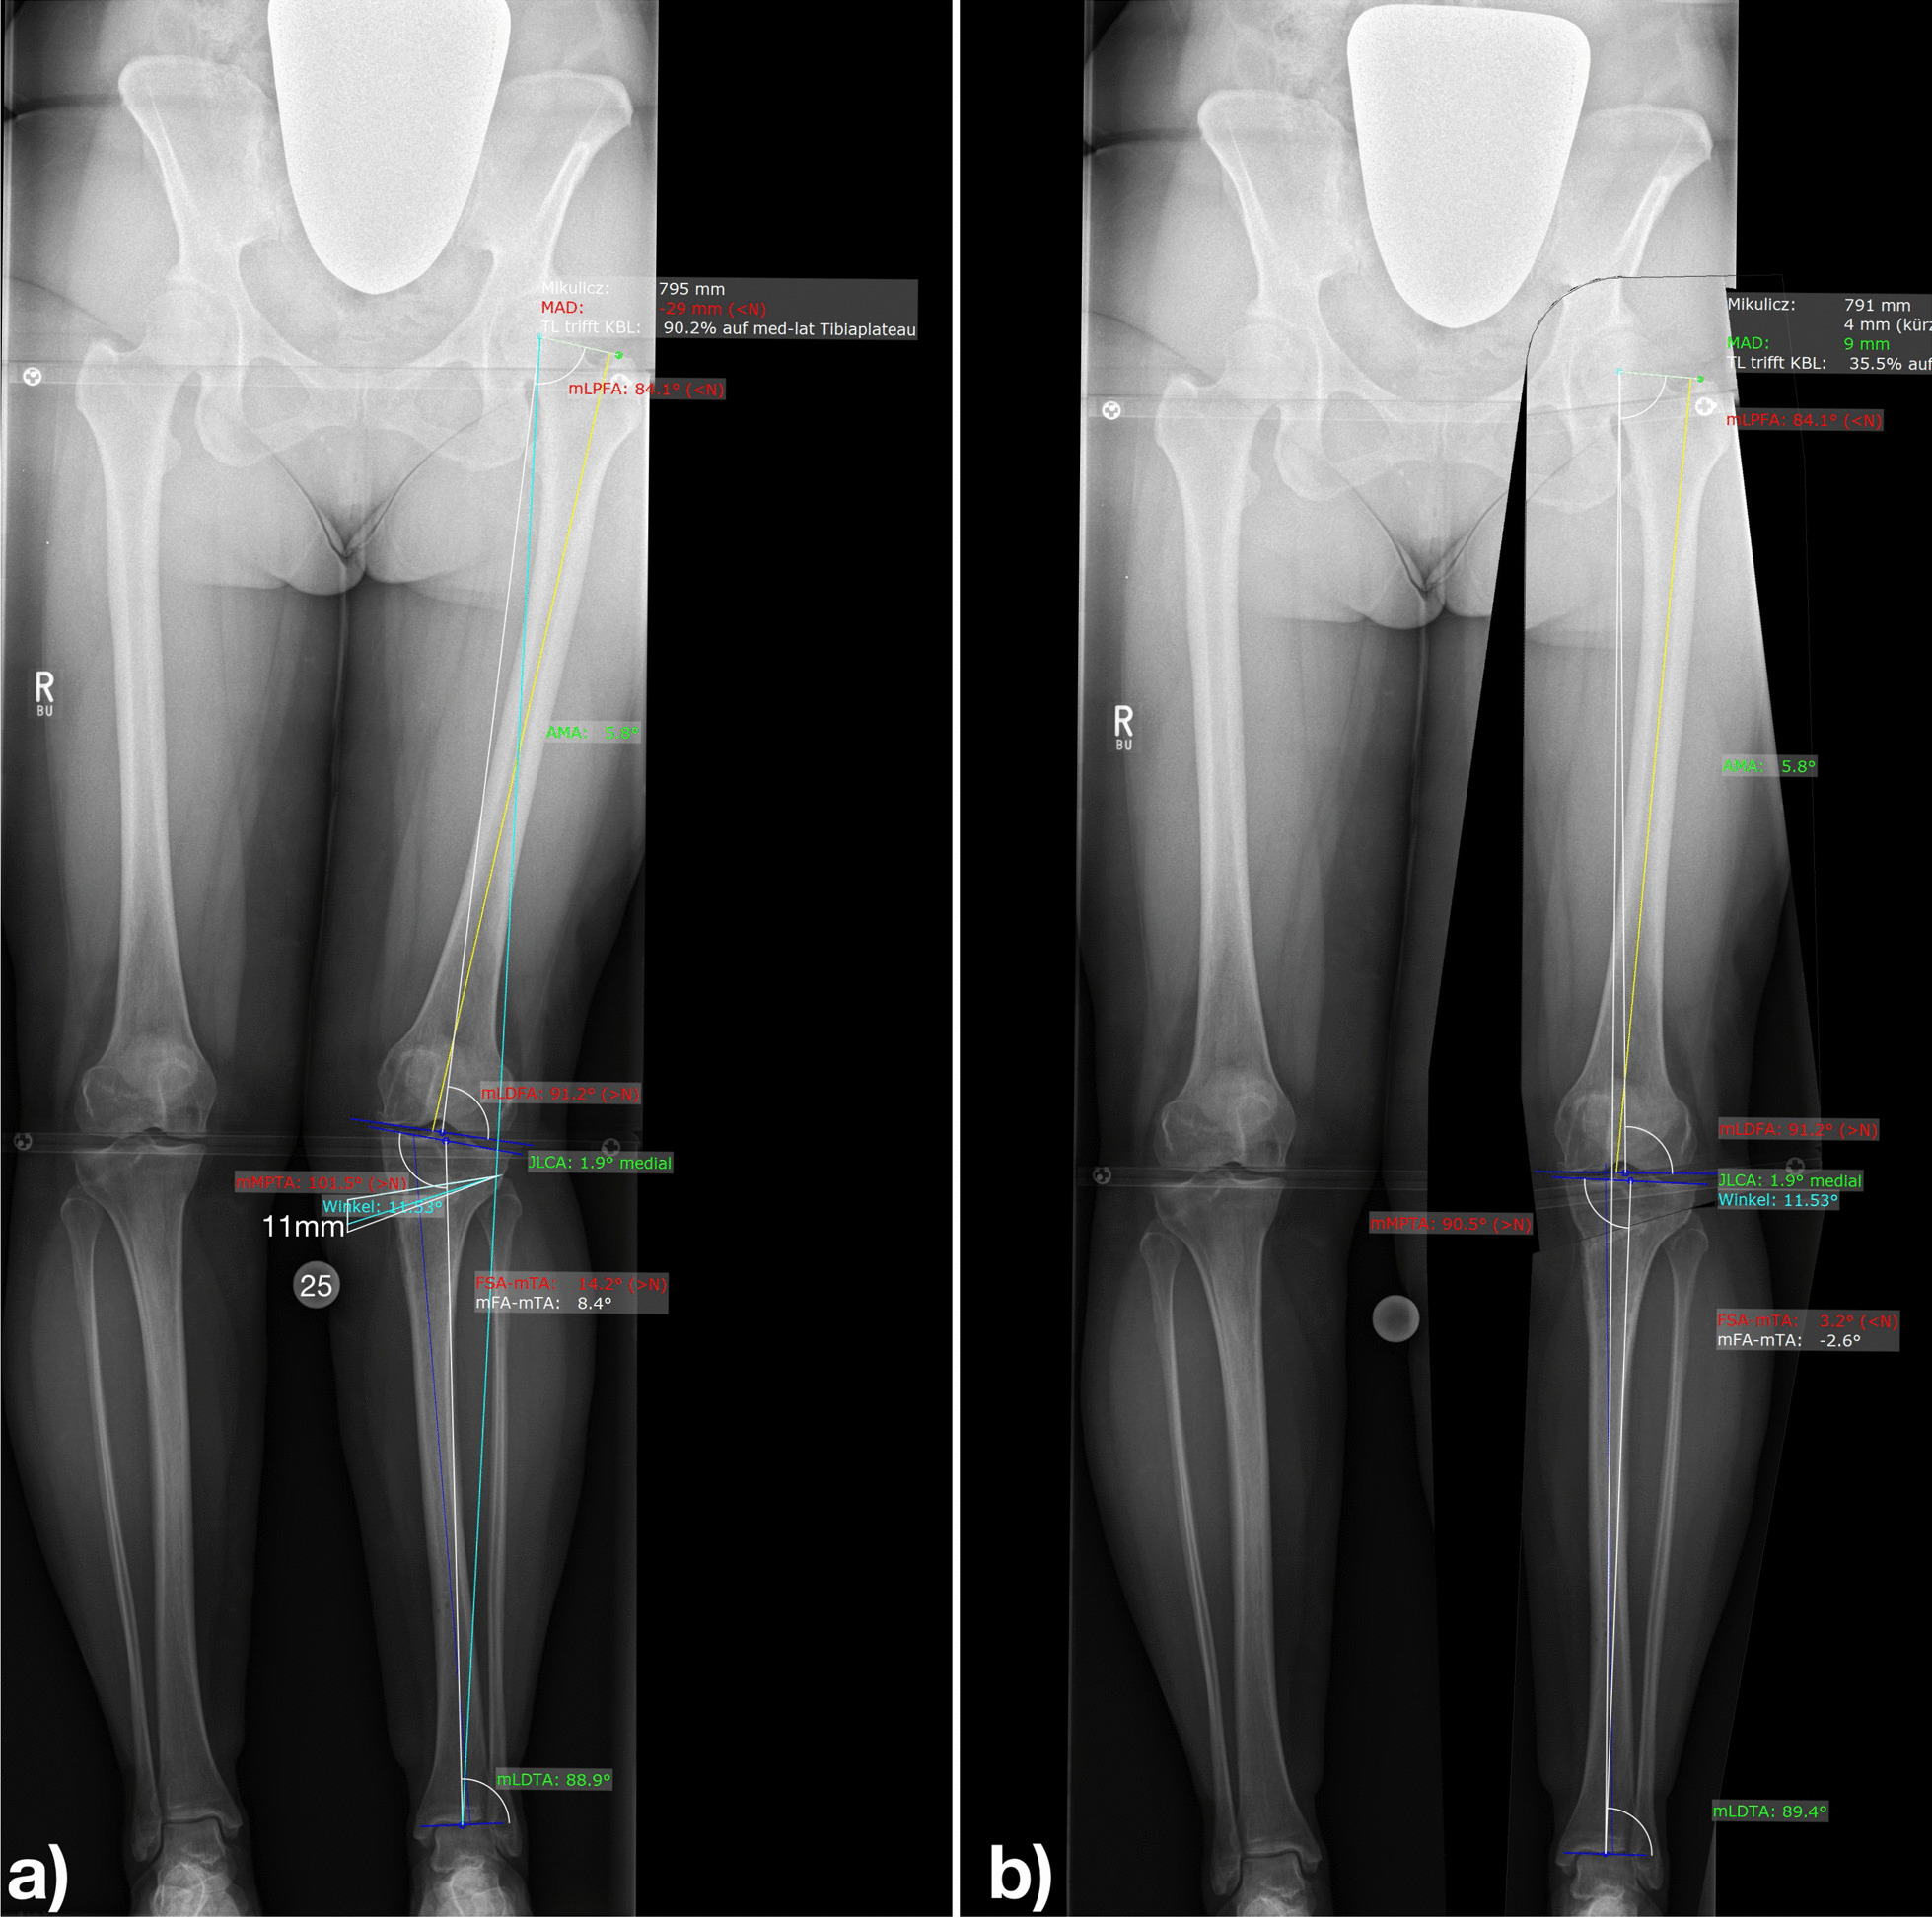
Long-leg standing radiograph. a Valgus aligned knee with oblique joint-line and minimal ischiofemoral space between the ischial bone and the lesser trochanter. Using mediCAD software, a medial closed wedge high tibial osteotomy for varization is planned on the native X-ray showing the planned surgical procedure (11-mm closed wedge high tibial osteotomy) and b the planned result after osteotomy
